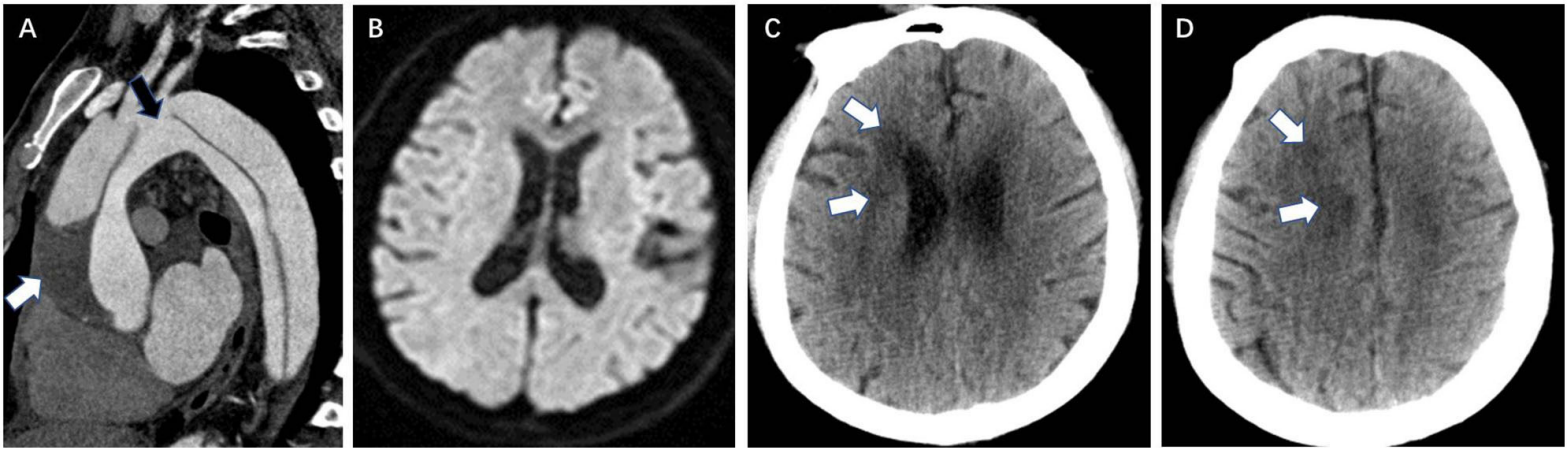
Retrograde aAO dissection with thrombosis of the false lumen is a CTA risk finding for postoperative new stroke in a 39-year-old man with ATAAD. (A) Oblique sagittal MPR image shows an entry tear (black arrow) in the aortic arch and the intimal flap extends into the aAO in a retrograde manner. Low-density thrombus (white arrow) is present in the false lumen due to the integrated intimal fap in the aAO. (B) Preoperative diffusion-weighted magnetic resonance images show normal brain. (C,D) Brain CT 13 days after surgery shows a new low-density ischemic infarction in the right frontal lobe, area around the right ventricular body, and centrum semiovale (white arrow). aAO, ascending aorta; CTA, computed tomography angiography; ATAAD, acute type A aortic dissection; MPR, multiplanar reformation.
